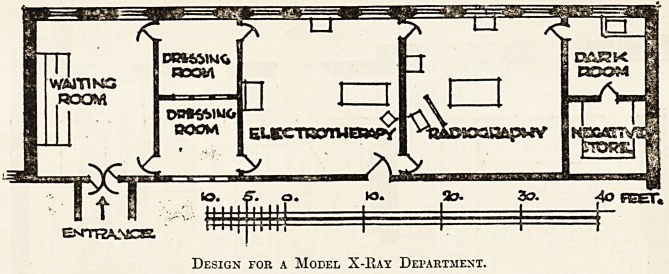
# Electro-Therapy and Radiography
